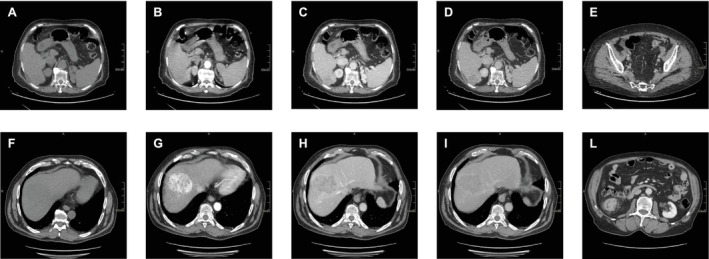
CT scans of patients with diagnosis of moderately differentiated trabecular HCC. Scans of case 1 patient showing HCC in basal (A), arterial (B), venous (C) and late (D) phases, and colon carcinoma (E), and scans of case 3 patient showing HCC in basal (F), arterial (G), venous (H) and late (I) phases, and colon carcinoma (L).
